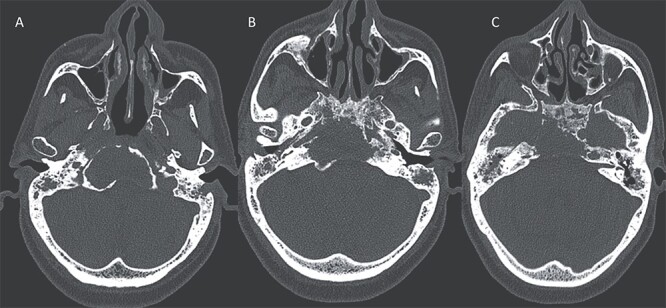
Axial CT in bony window showing: (A, B) extensive bone erosion of the clivus and the body and right greater wing of the sphenoid. (C) Soft tissue in the middle ear cavity and mastoid cells.
